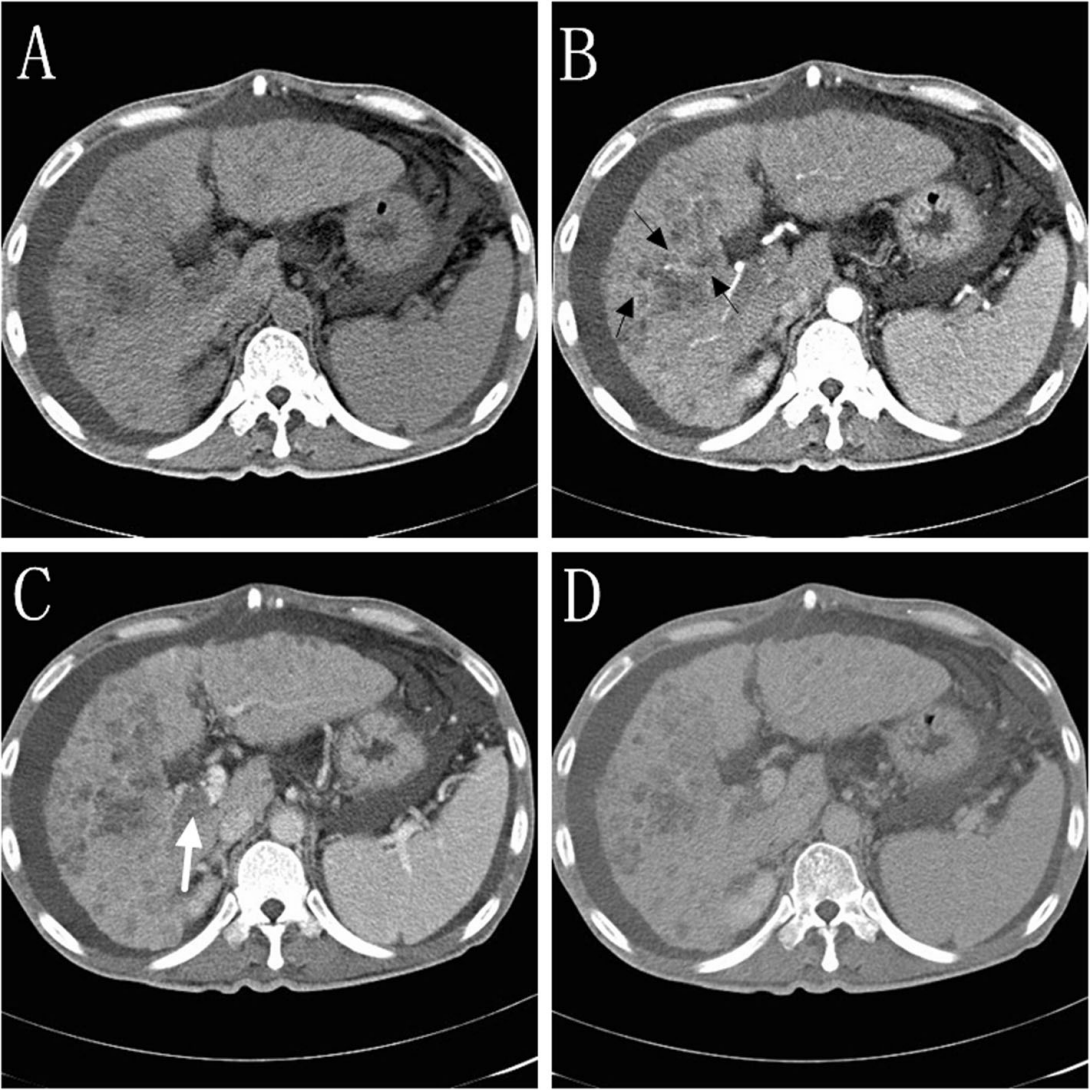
Images of a patient with infiltrative HCC (iHCC). Unenhanced CT image showing a low-density lesion in the right lobe of the liver (a), contrast-enhanced image showing the lesion slightly heterogeneously enhanced in the arterial phase with a “mosaic sign” (black arrow) (b); “washout” performance with tumor thrombus is noted in the right branch of the portal vein (white arrow) in the portal venous phase (c) and delayed phase (d)
